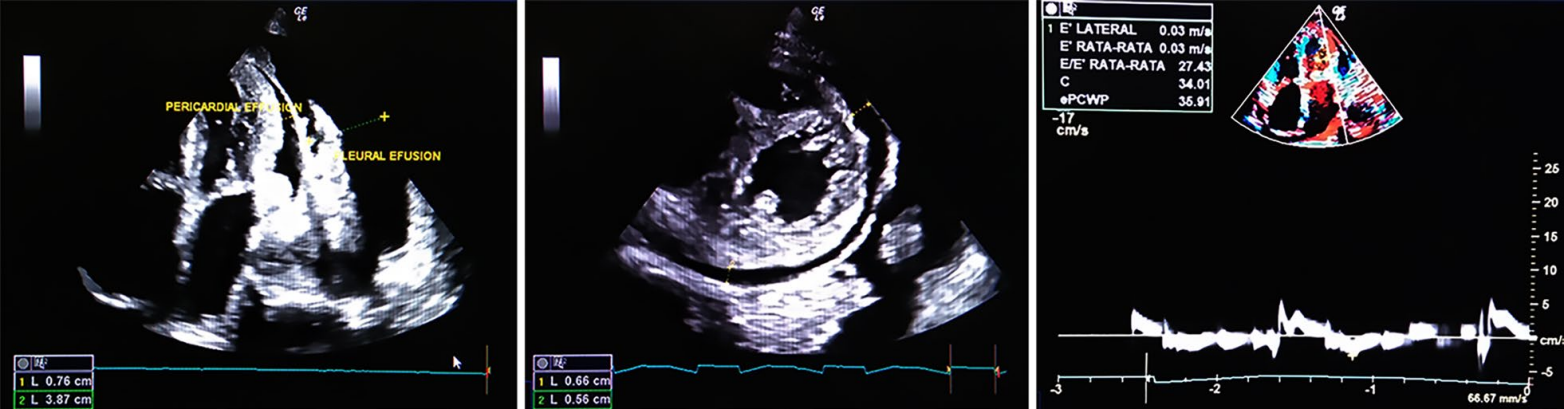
Transthoracic echocardiogram displayed increased left ventricular filling pressure with diastolic dysfunction grade III, mild circumferential pericardial effusion with adjacent pleural effusion
